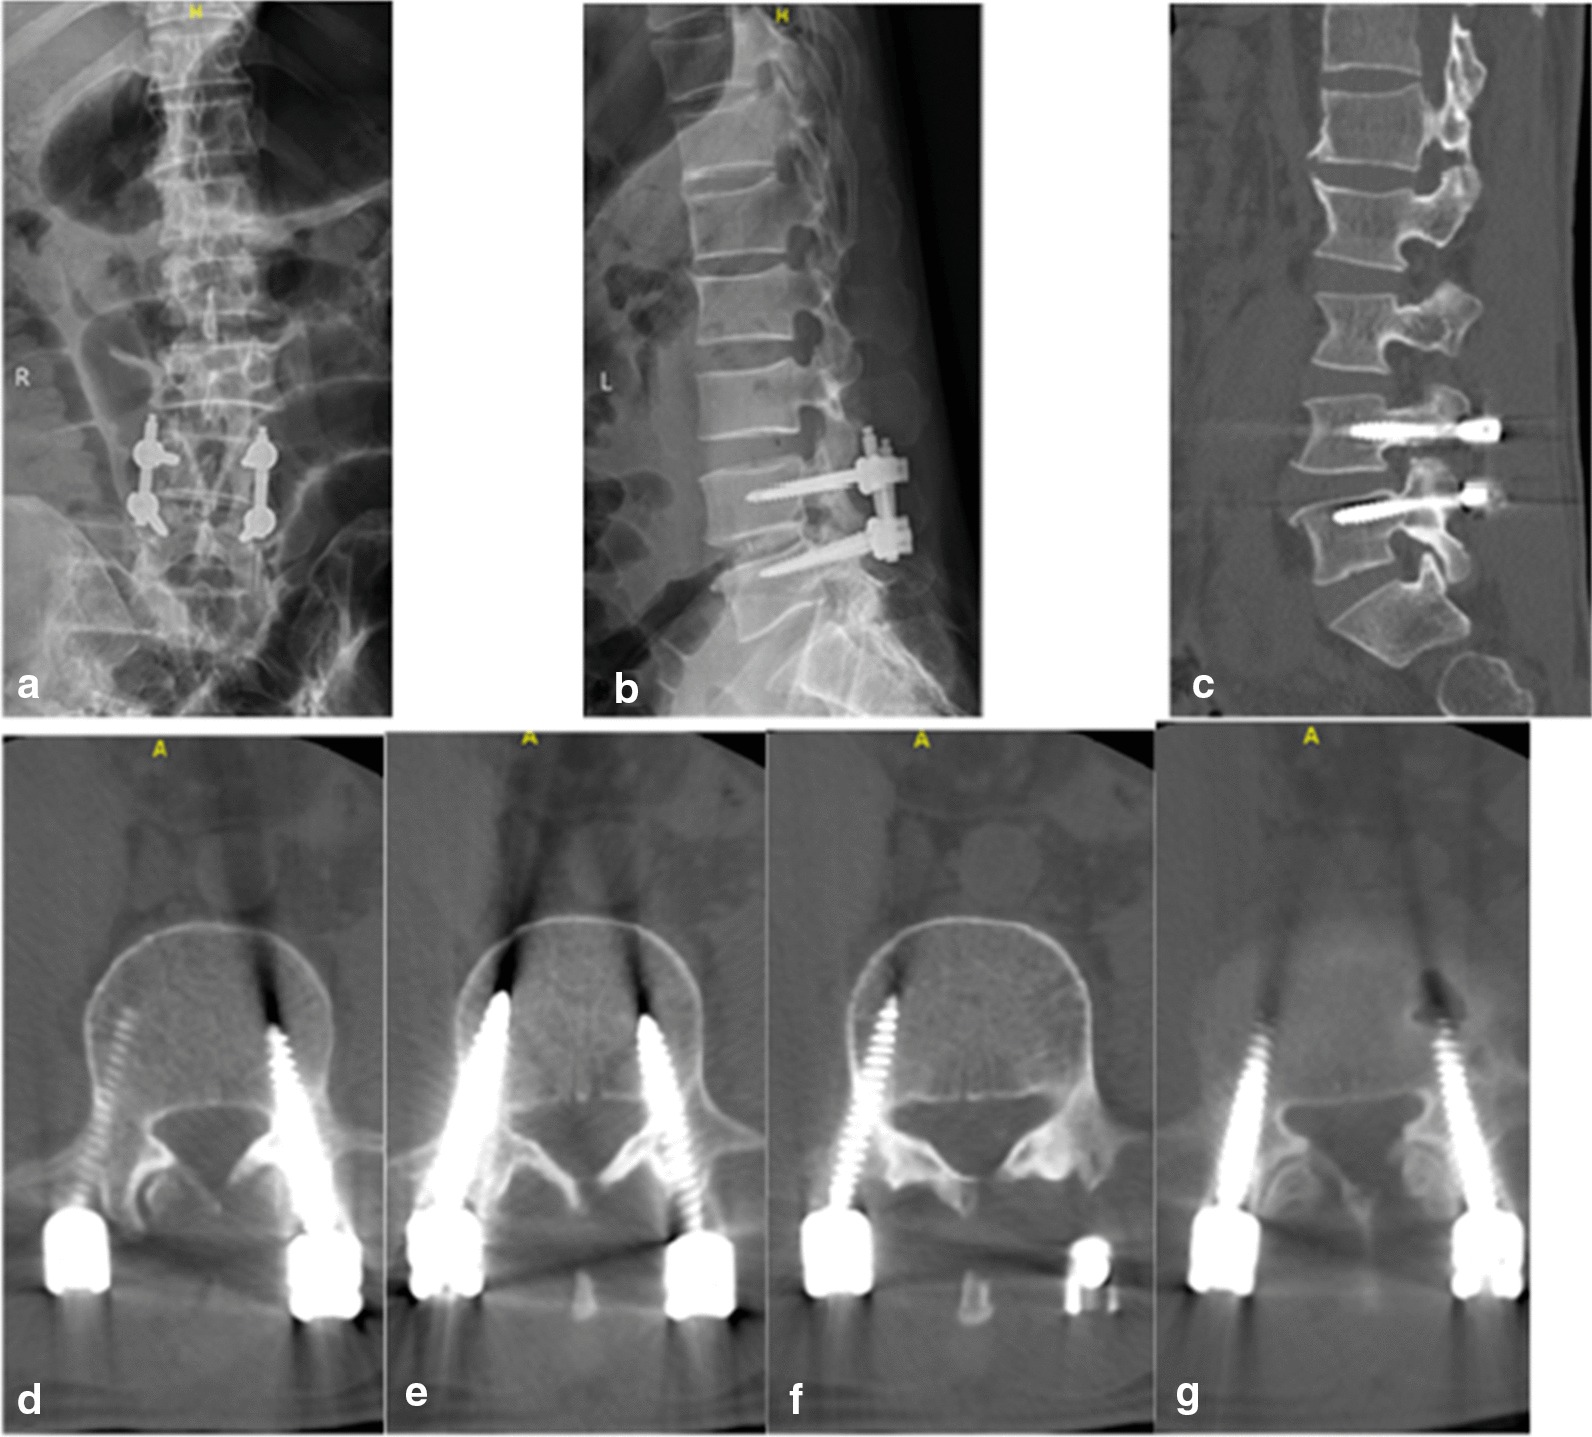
Lumbar CT and X-ray examination 20 days post-surgery. a, b Anterior lateral radiograph of the patient's lumbar X-ray. c–g Sagittal and transverse sections of radiograph of the patient's lumbar CT
